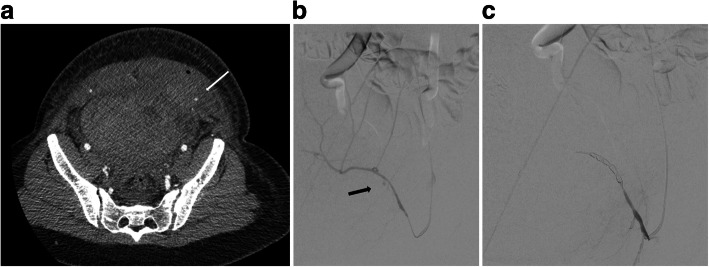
a-c Haemorrhage and embolisation due to PSA bleeding. a CT shows abdominal wall haematoma with small pseudoaneurysm. b Angiography from a contra-lateral approach with 4F cobra catheter and microcatheter use, confirms small false aneurysm arising from the inferior epigastric artery. c superselective embolisation with multiple 2 and 3 mm micro coils
